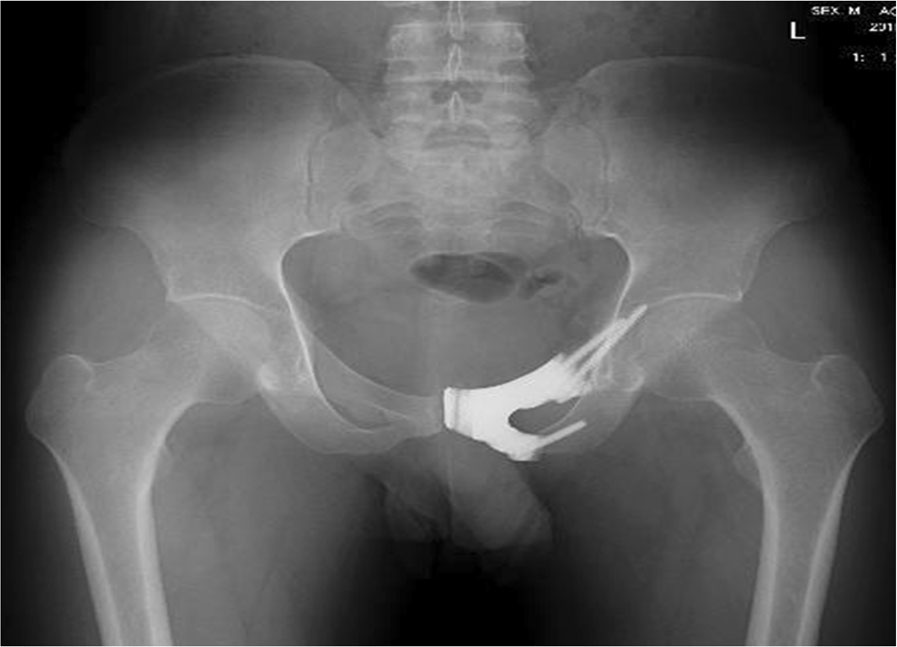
Two months after the operation, X-ray showed well alignment of prosthesis
